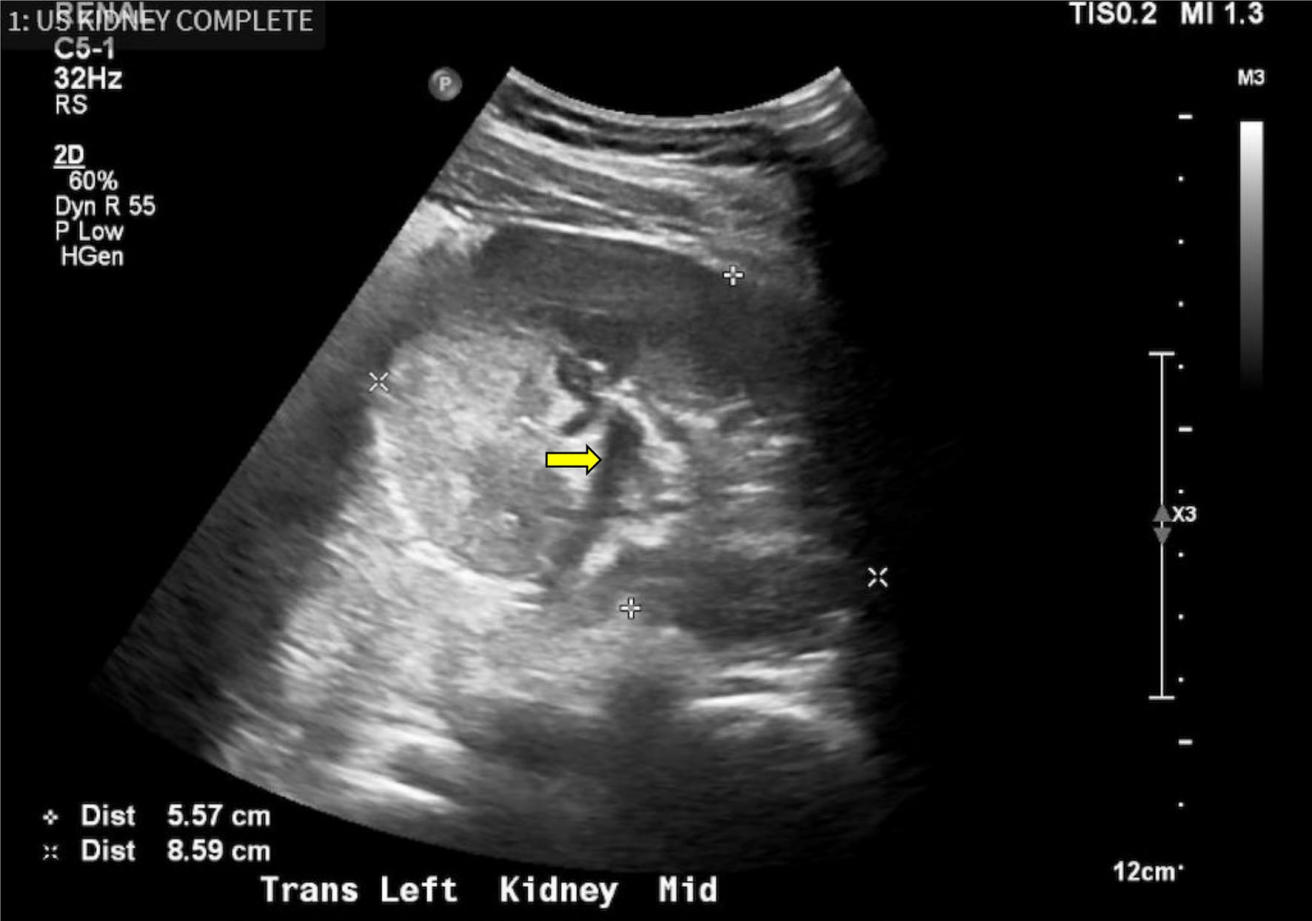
Transverse ultrasound of the left kidney (axial view)
